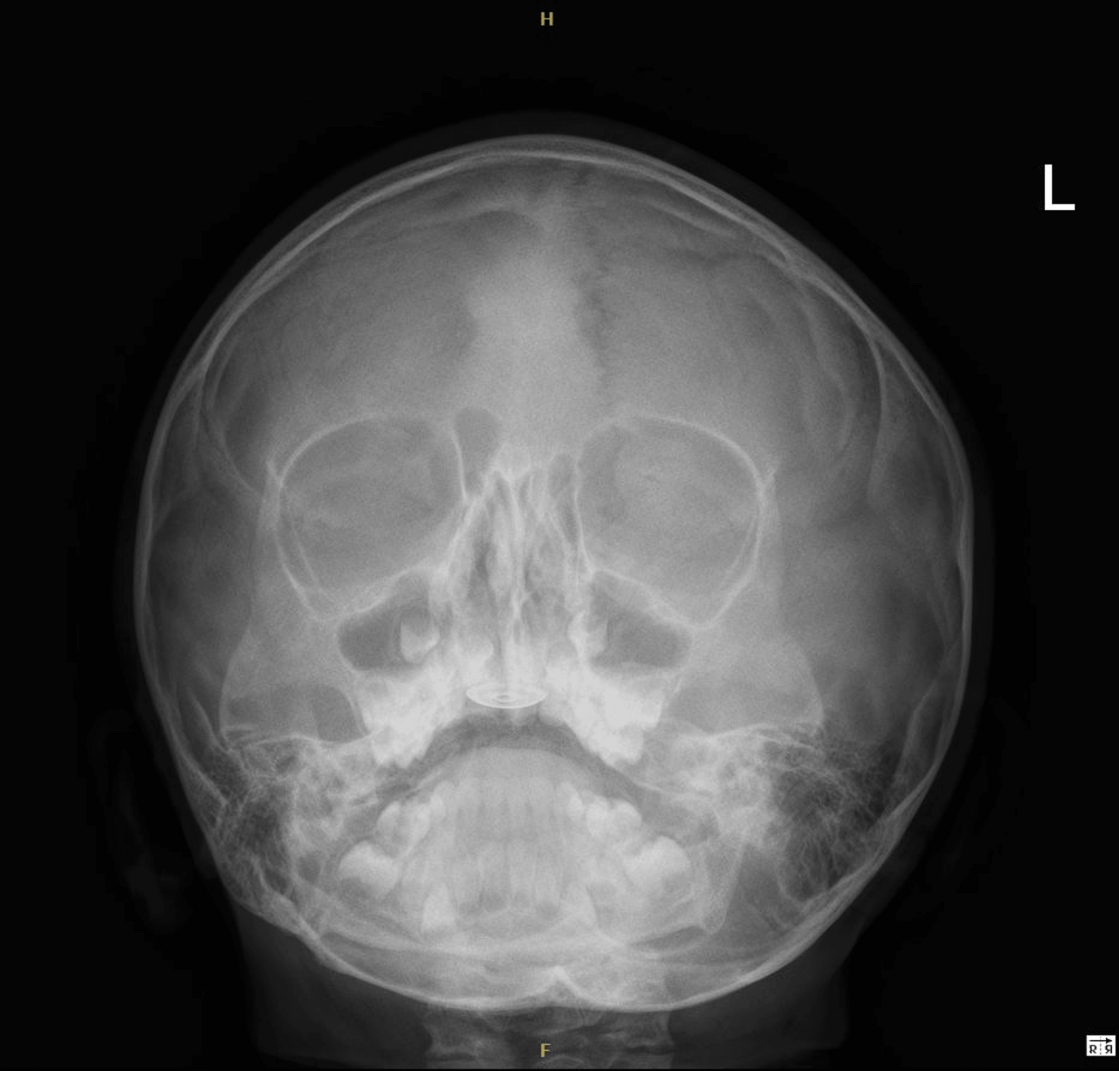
X-ray showing the flat foreign body. Skull X-ray, paranasal sinuses Waters view showing a round and flat radiopaque foreign body on the hard palate.
